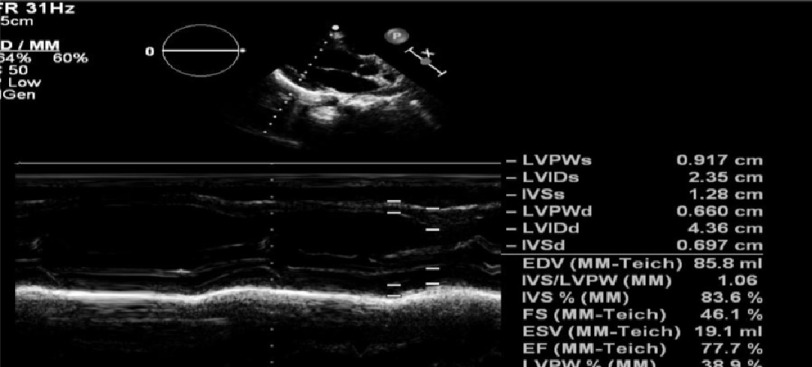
Parasternal long axis view: normal LV dimensions with normal systolic function.
